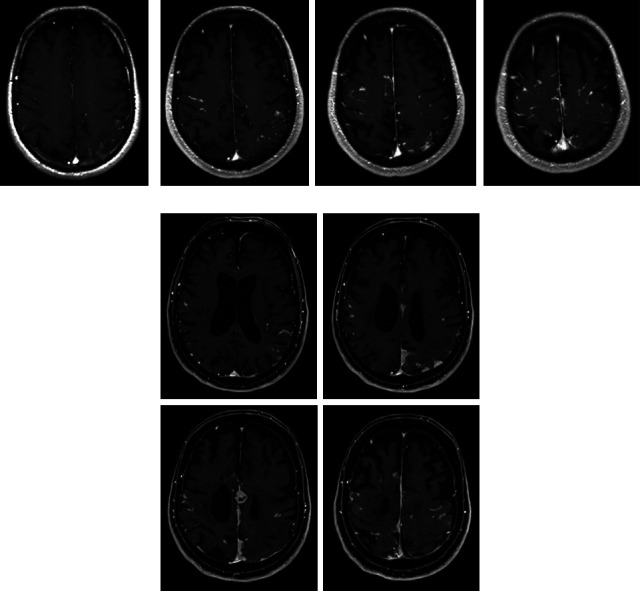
Axial T1 (longitudinal relaxation time) magnetic resonance imaging of the brain with contrast in (a) January 2008, (b) April 2010, and (c) January 2020, showing progression of multiple heterogeneously enhancing dural base and leptomeningeal lesions.
